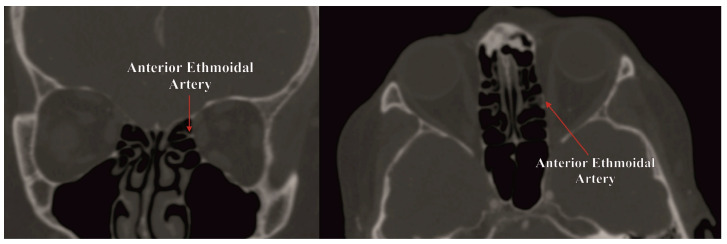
Anterior ethmoidal artery observed through computed tomography angiography—coronal and axial views.
